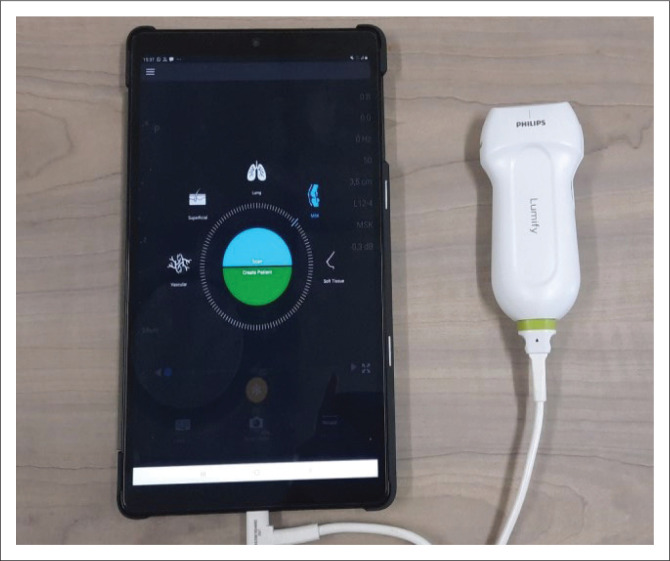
Handheld transducer attached to portable device displaying ultrasound application.
